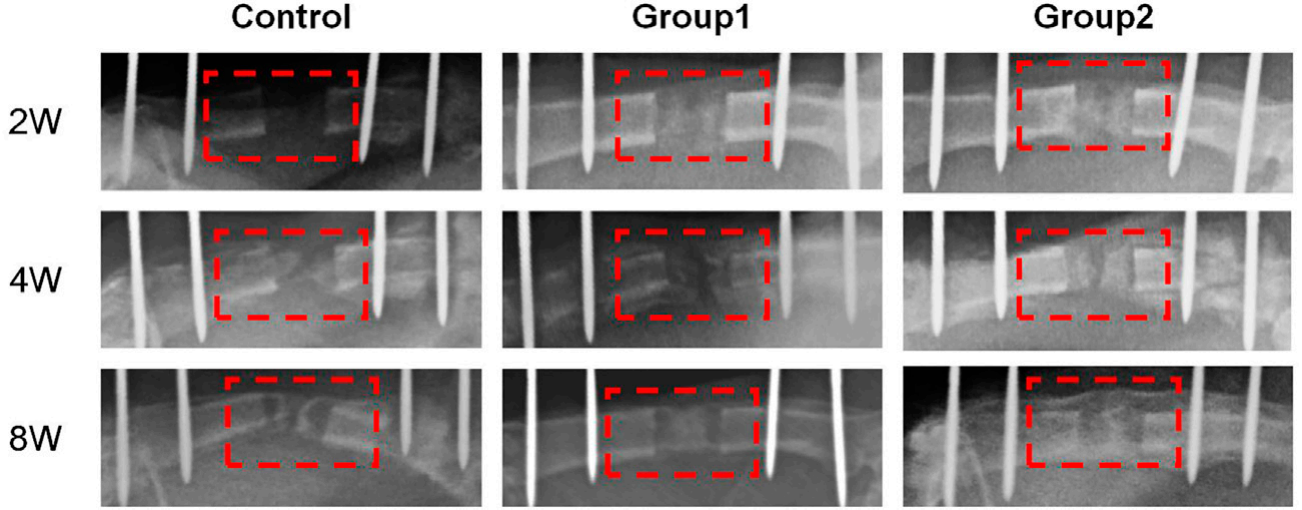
X-ray photos of femoral samples at different time points.
